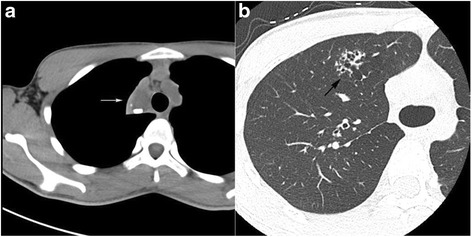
a Plain chest computed tomography image (axial) shows a right anterior mediastinal cystic mass with calcification (*arrow*). b Chest computed tomography image shows a cystic lesion in the right anterior segment (*arrow*)
